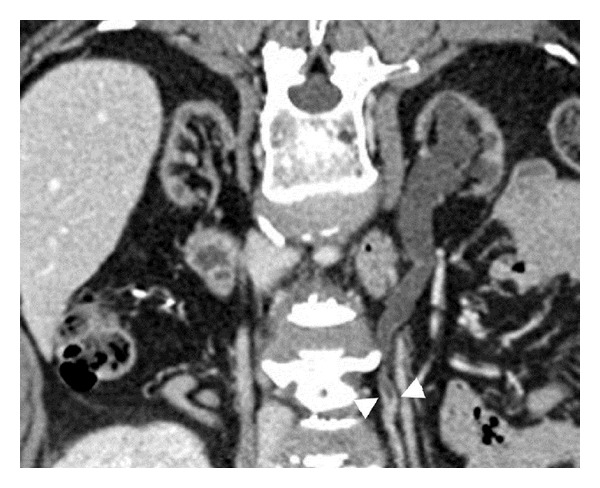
Curved planar reformatted image of computed tomography urography of a 56-year-old female kidney transplant recipient exhibits a fork sign (arrowheads) in the left native ureter representing a left ureteral UC.
